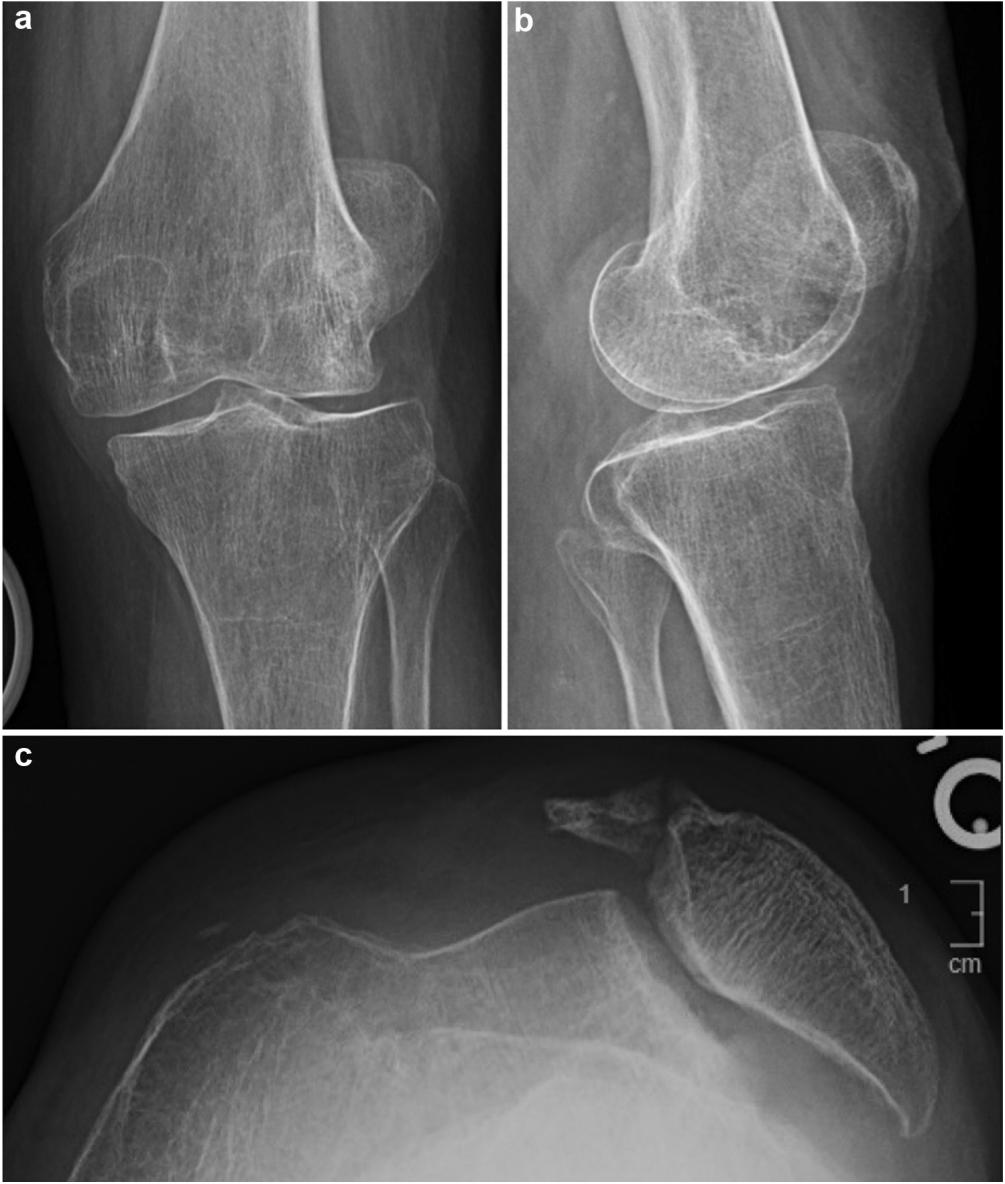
Preoperative X-rays of the left knee. Anteroposterior (a) and lateral (b) views demonstrate osteoarthritis with lateral dislocation of the patella. Merchant view (c) shows laterally dislocated patella.
